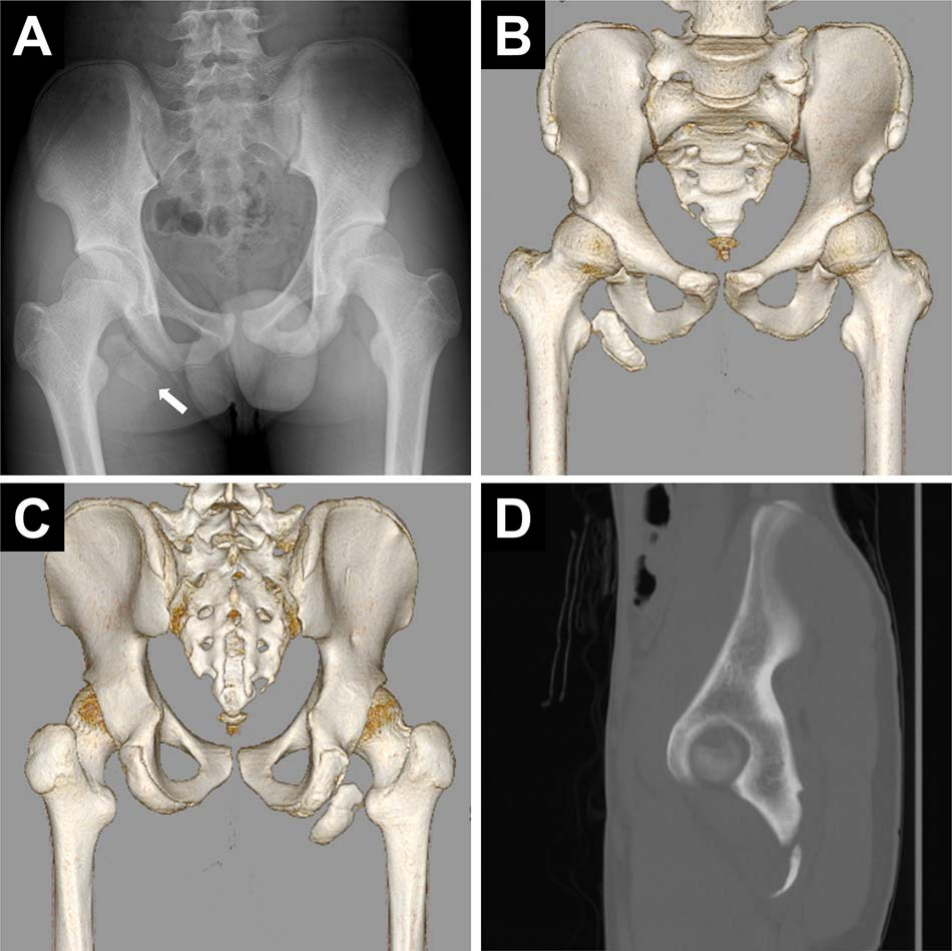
Preoperative images of the pelvis. (A) Pelvic radiograph showing the displaced avulsion fracture of the ischial tuberosity (white arrow). (B, C) Computed tomography scans show the displaced avulsed fragment. The avulsed fragment was displaced by 35 mm. (D) The size of the avulsed fragment was 55 × 17 mm.
